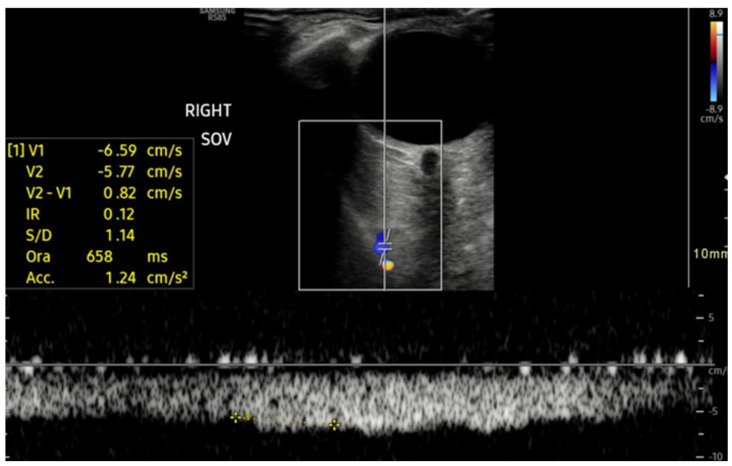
Superior ophthalmic Vein (SOV) courses in the superior part of the orbit and is usually depicted in blue, with a Doppler signal characterized by a continuous venous flow with maximum and minimum velocities (maxV and minV) typically less than 10 cm/s; in some cases, the Valsalva maneuver may be useful to improve visualization.
